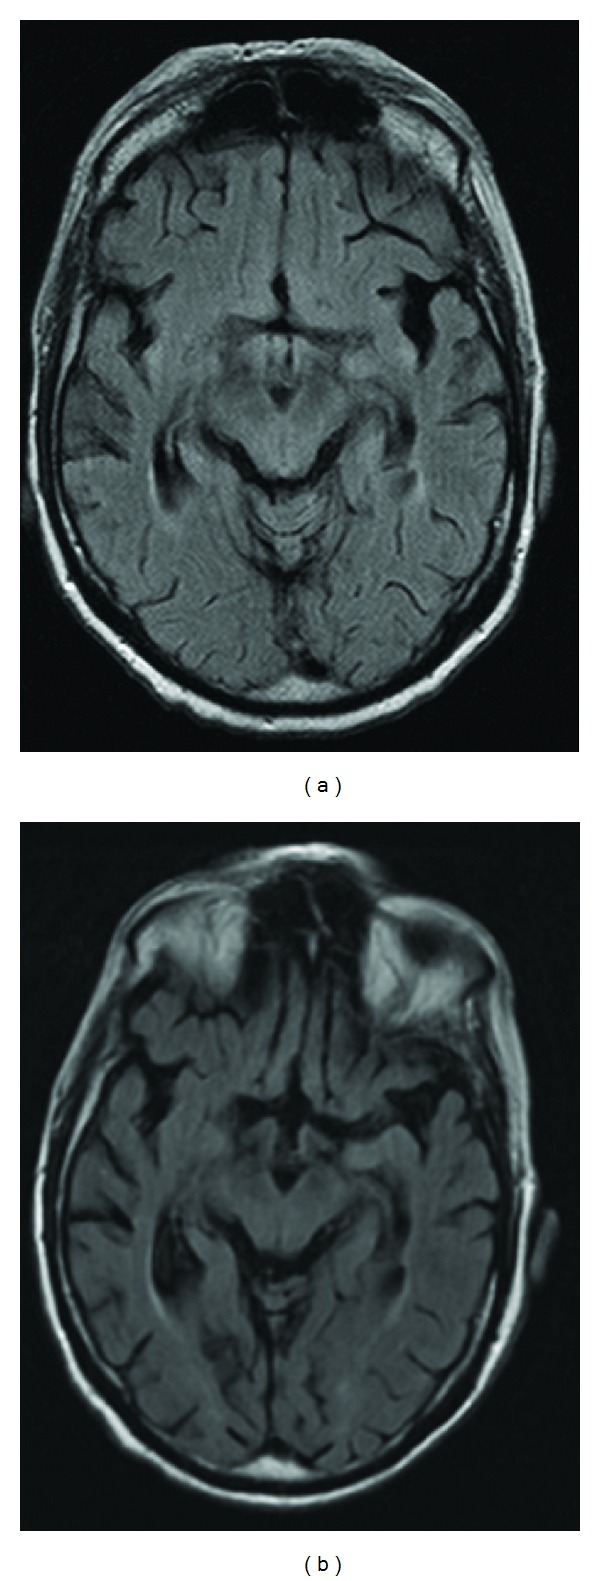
Axial FLAIR images obtained before initiation of Rituximab. Normal anatomy and signal intensity of limbic system were seen at initial presentation as well as after longer course of disease (left: November 2007; right: February 2008).
